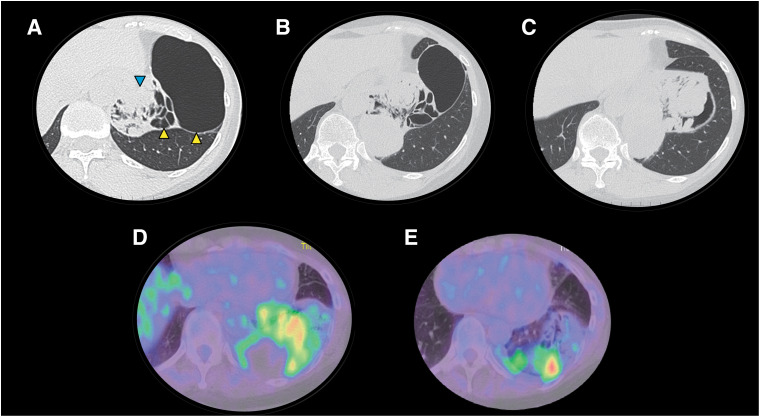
Radiological changes to the tumor and pulmonary cyst in the left lower lobe. (A) A chest CT scan at the previous hospital revealed a multifocal cyst (yellow arrowhead) and cyst wall nodule areas (blue arrowhead). (B) The cyst had shrunk 3 years after being detected. (C) The cyst had grown to a diameter of 10 cm with fluid effusion and progressive enlargement of the cyst wall 4 years after being detected. (D, E) FDG-PET/CT performed 5 years after detection revealed an SUVmax of 5.2 for the solid component of the cystic tumor, but slight accumulation in the fluid or cystic septum. CT: computed tomography; FDG-PET/CT: ^18^F-fluorodeoxyglucose-positron emission tomography/computed tomography; SUVmax: maximum standardized uptake value
